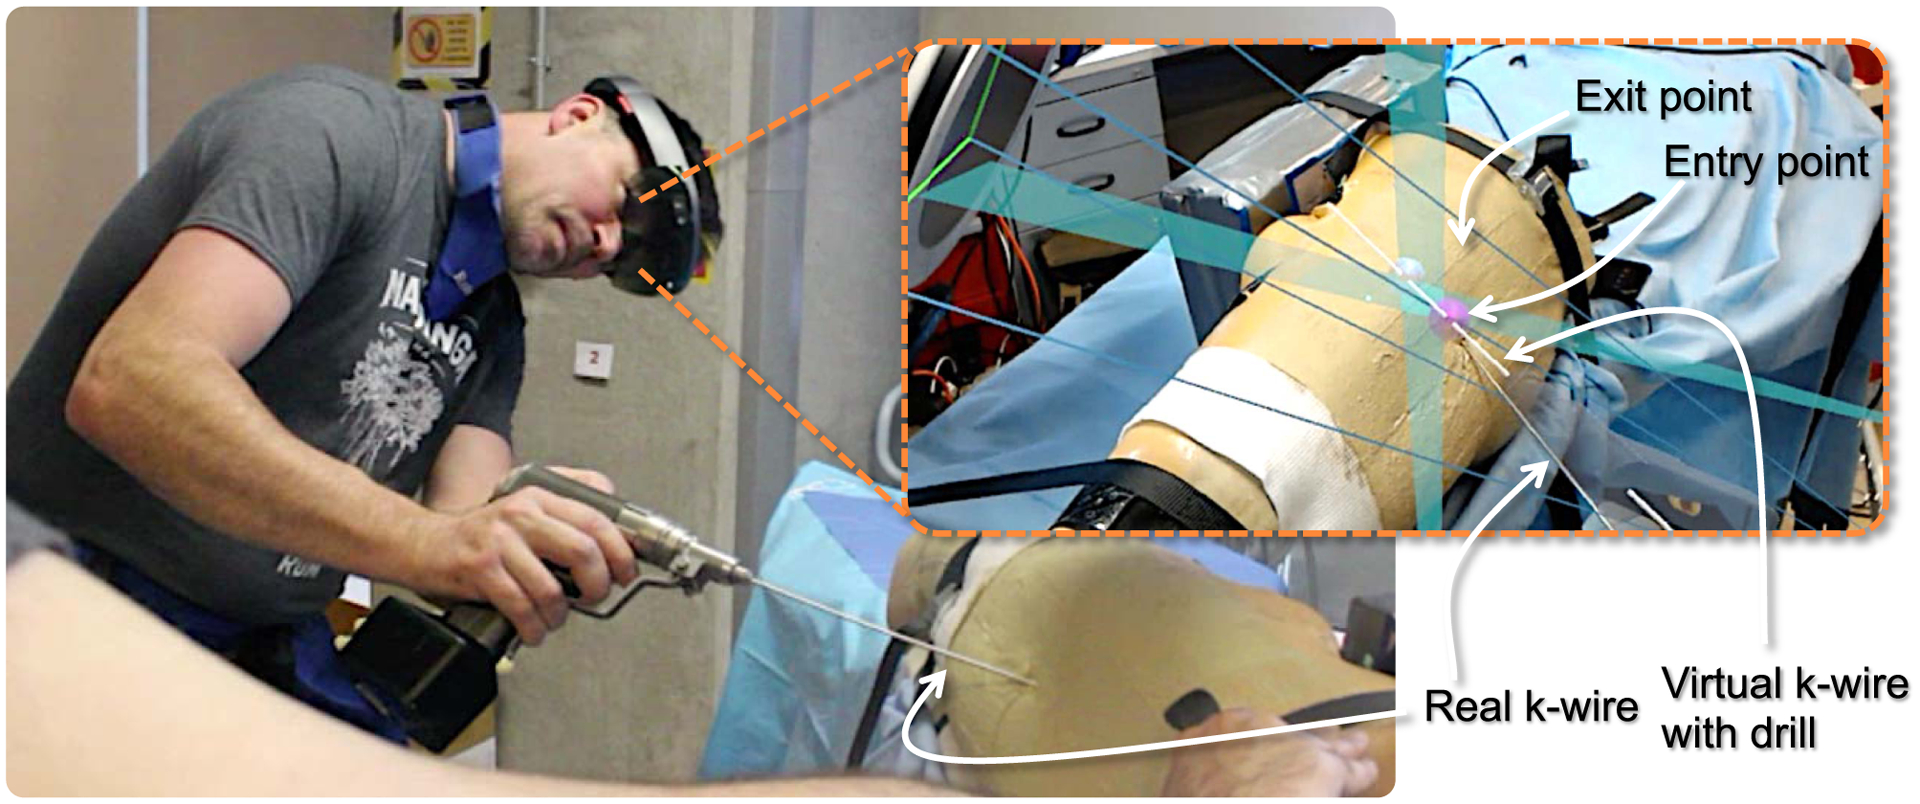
In K-wire placement for fracture reduction procedures, the surgeon can plan the entry and exit points of the wire on two X-ray images. After the planning, two triangular planes are constructed by connecting the drilling trajectory defined on the detector plane (X-ray image) and the C-arm source (X-ray origin). The intersection of these two planes is a line that corresponds to the desired drilling trajectory in 3D. By exploiting the imaging frustum, this line is augmented directly on the patient anatomy. The surgeon can then align the physical drill with its virtual counterpart, and advance the wire through the anatomy.
